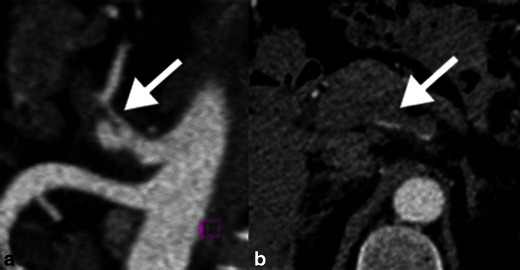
Spontaneous coeliac artery dissection. a Contrast-enhanced sagittal reformatted image demonstrates a dissection flap of the coeliac artery (*white arrow*). b Axial CT image shows the dissection flap continues into the common hepatic artery with thrombosis of the false lumen. There was no evidence of bowel ischaemia in this patient and the appearance remained stable on follow-up imaging
